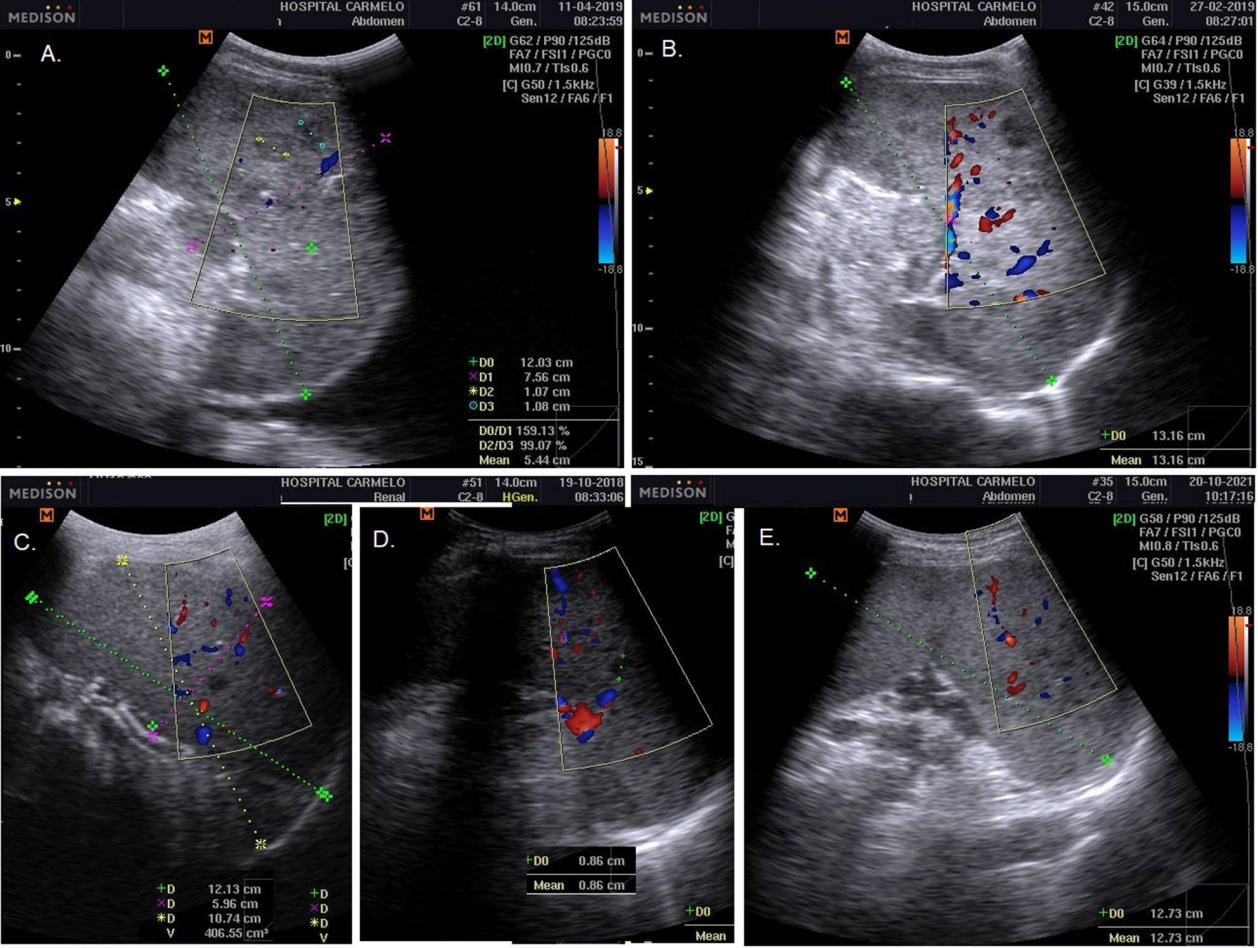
Splenic TB: macronodular pattern characterized by splenomegaly with single or multiple, hypo(anechoic) macronodular lesion; color doppler with hypo flow, compatible with focal caseous splenic lesion (A–C); and miliary pattern characterized by a splenomegaly with hypo(anechoic) diffuse multiple nodular lesions (D, E).
